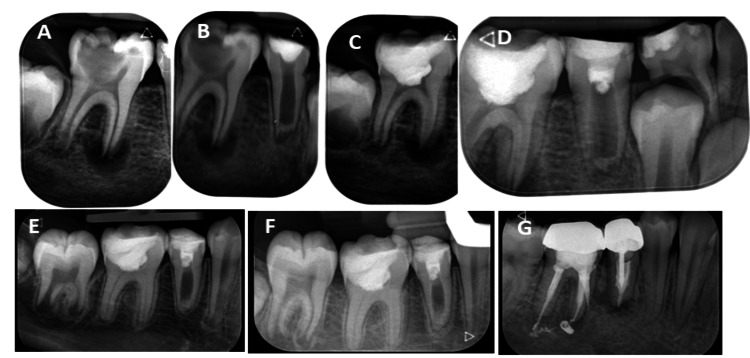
Case 1 - radiographic images (A) Radiograph of tooth #30 upon first arrival at the office. (B) Radiograph of tooth #29 upon first arrival at the office. (C) Radiograph of tooth #30 at the end of vital pulp therapy. (D) Radiograph of tooth #29 at the end of regenerative endodontic treatment. (E) Follow-up radiograph at 28 months. A traceable periodontal ligament along both roots of tooth #30 and more dentin in the apical area of the canal of tooth #29 were noted. (F) Follow-up radiograph at 46 months. A traceable periodontal ligament along both roots of tooth #30 and more calcified tissue in the pulp of tooth #29 were noted. (G) Follow-up radiograph at 100 months. Teeth #29 and #30 were treated with root canals and crowns.
